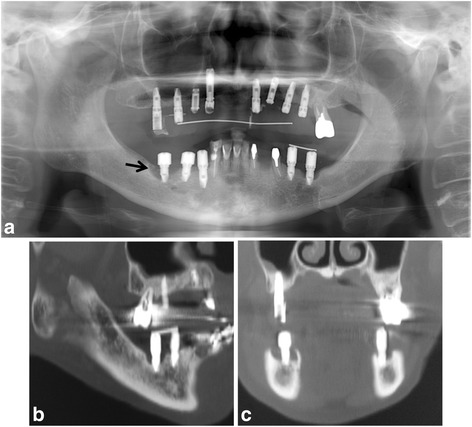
a Panoramic radiograph showing marked alveolar bone resorption surrounding the dental implant replacing the right mandibular first molar (*arrow*). b Sagittal CT view. c Coronal CT view
